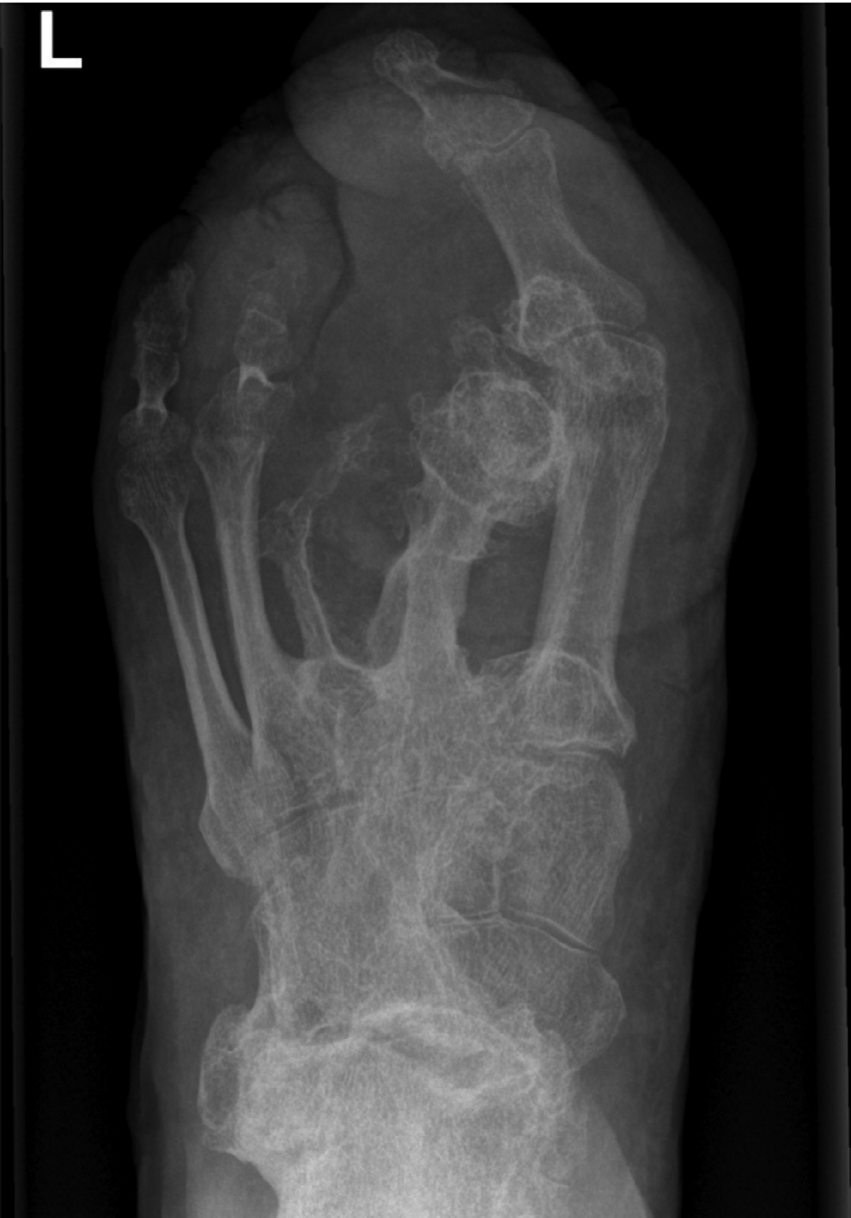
Conventional A-P radiograph of the left foot of case 1 showing a synostosis between metatarsal I and II. Status after amputation of the first and second toe in 1962 and additional soft tissue debulking surgery in 1964, 1965, 1973, and 1978.
